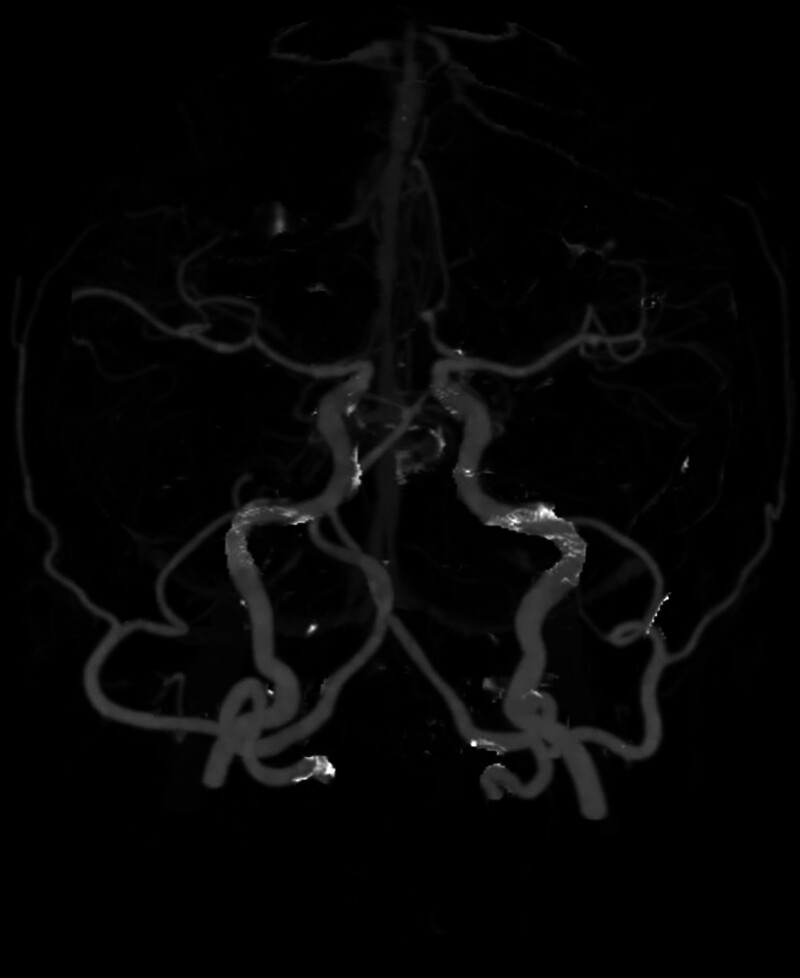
Cranial CTA findings were as follows: a slightly thickened lumen of the anterior communicating artery; a hummocky shadow with smooth margins at the cavernous segment of the right internal carotid artery; variation of the triploid anterior cerebral artery; and a bilateral tortuous V4 segment vertebral artery. Aneurysms cannot be excluded. CTA = computed tomography angiography.
